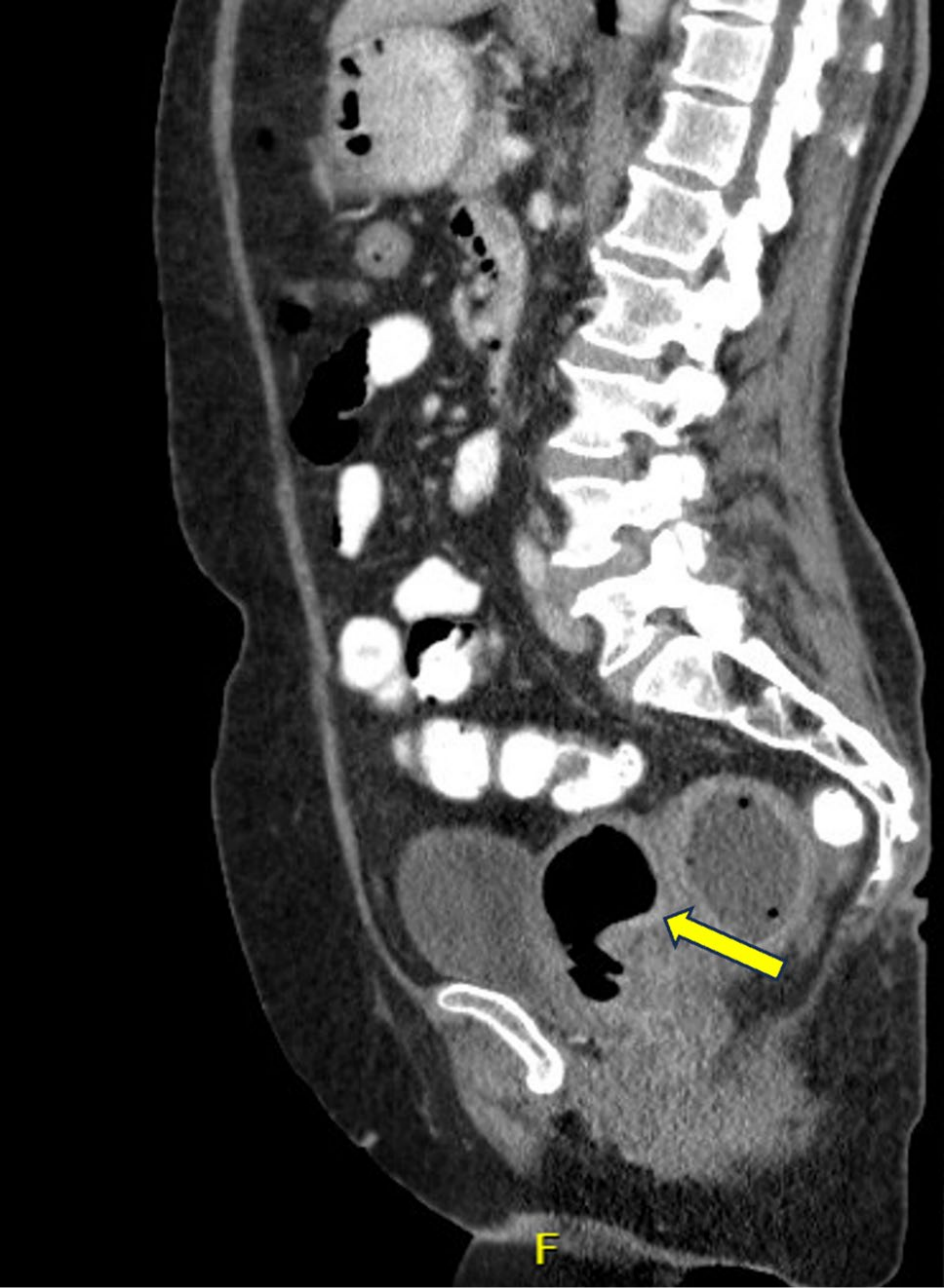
Sagittal CT scan demonstrating a retroflexed uterus with distension of the uterine fundus by gas and fluid
